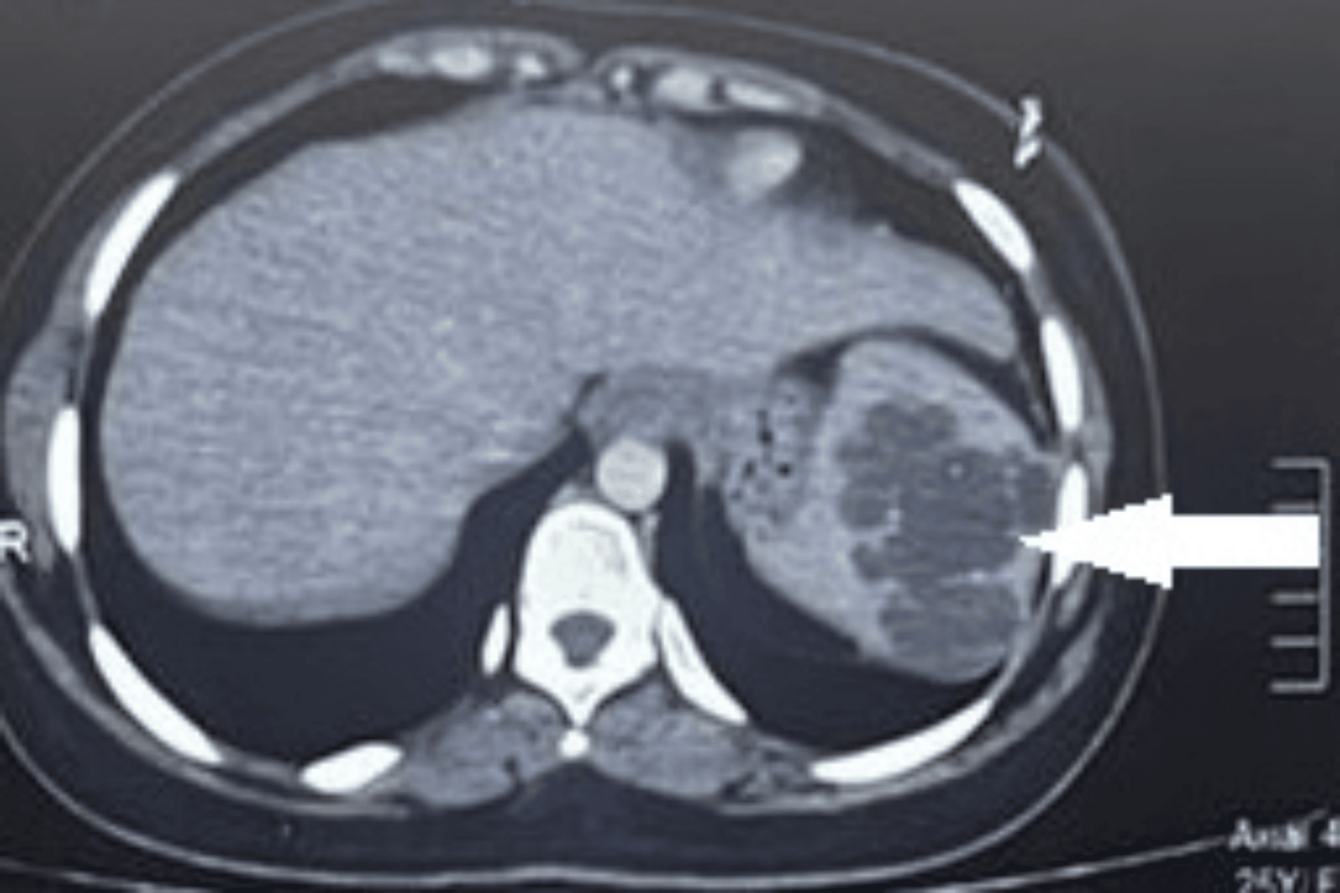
Primary splenic cyst Contrast enhanced CT abdomen image showing a multiloculated cyst in the superior pole of the spleen (white arrow).
